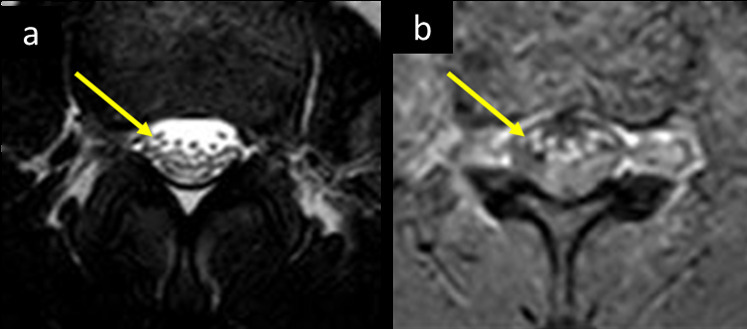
A 25-year-old male presented to the ED with upper and lower limb weakness which progressed gradually over the past 2 days, associated with pain in the upper neck region. He was also complaining of fever and diarrhea for the last 5 days, with no respiratory symptoms. He tested positive for COVID-19. His neurological examination showed power of 3/5 in all four limbs, which got worse to become 1-2/5 in all limbs in the subsequent days. He was started on IVIG with mild improvement of the lower limb weakness over next 5 weeks. MRI at upper lumbar and cauda equina level (a) axial *T*_2_ and (b) *T*_1_WI post-i.v. contrast demonstrates thickening and post contrast enhancement of intradural ventral nerve roots, consistent with Guillain-Barre syndrome (Arrows in a and b). ED, emergency department;IVIG, i.v. immunoglobulin.
